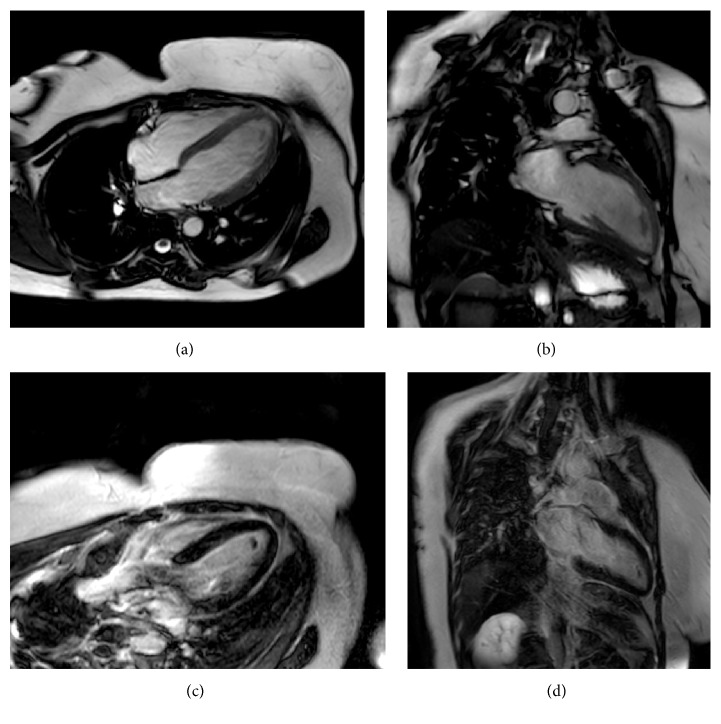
Cardiac magnetic resonance imaging showing a decreased thrombus size in two months following ALP poisoning (after anticoagulant therapy). (a) Four-chamber view and (b) two-chamber view show a decrease of thrombus size of 80%; (c) four-chamber view (10 min of late gadolinium enhancement): regression of LGE in inferoseptal and anterolateral walls and in the apex; (d) two-chamber view (10 min of late gadolinium enhancement): regression of LGE in the anterior wall.
